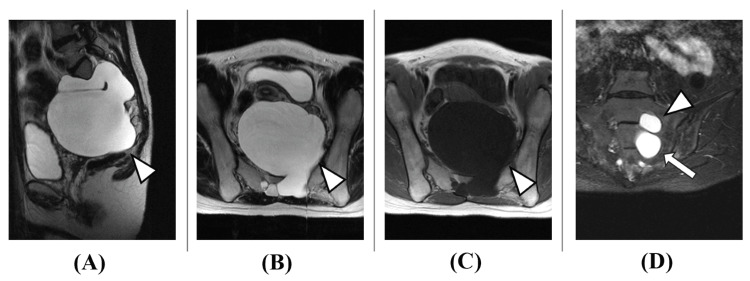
Initial pelvic MRI (A) T2 sagittal, (B) T2 axial, (C) T1 axial, (D) T2 coronal A cystic mass with a maximum diameter of 11 cm occupying the pelvic cavity was observed. The contents appeared as a uniform fluid collection with MRI T2 high intensity (arrowheads in A, B) and MRI T1 low intensity (arrowhead in C). A perineural cyst originating from the S2 (arrowhead in D) and S3 (arrow in D) nerve roots was suspected.
